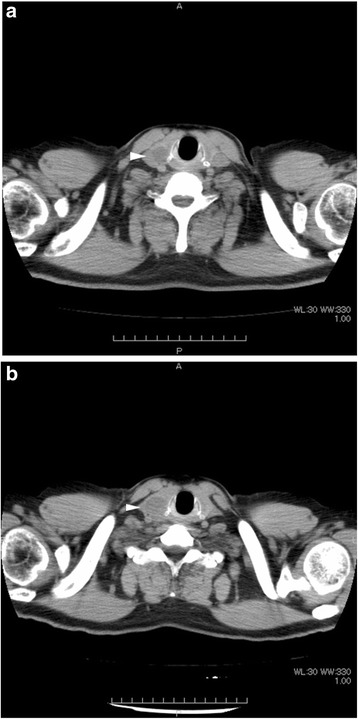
Computed tomography after two and half years (a) and three and half years (b) after definitive surgery for primary tumor. The white arrowhead indicates a growing low-density area in the right lobe of the thyroid gland
